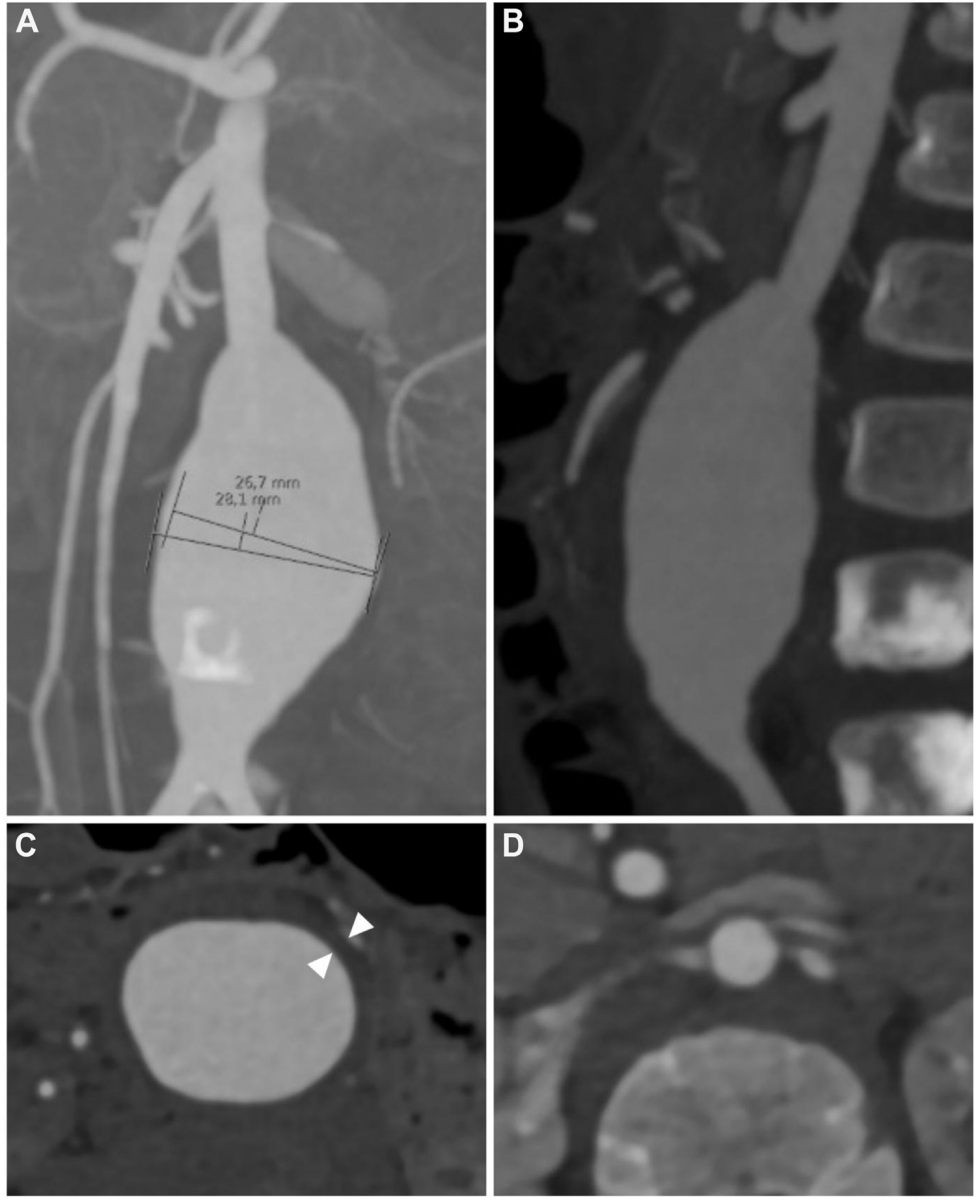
Computed tomography angiography demonstrating a 28-mm-wide infrarenal abdominal aortic aneurysm in a 2-year-old boy. Frontal view (A), sagittal (B), and axial views of the aneurysm (C) and the neck (D). Note the massive thickening of the aneurysm wall (C; *arrowheads*) in comparison with the normal infrarenal aorta (D).
